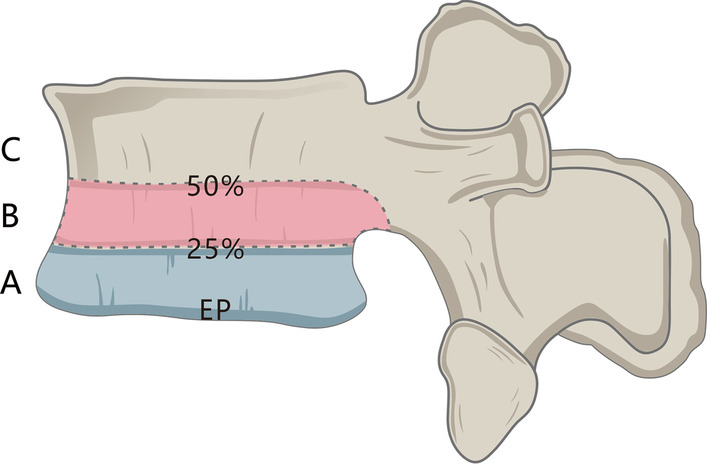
For grading according to the Modic changes in the height of the involved vertebral body on MRI, < 25% is grade A, between 25 and 50% is grade B, and > 50% is grade C
